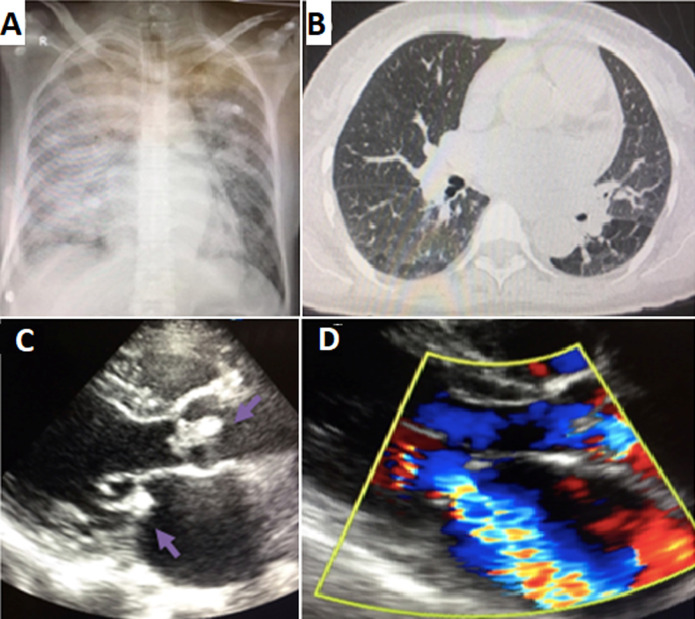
clinical case 2; A) chest X-ray showed a bilateral diffuse alveolar syndrome and cardiomegaly; B) thoracic CT scan a cardiomegaly with the presence of peripheral, central and bilateral ground glass lesion; C) transthoracic echocardiogram: parasternal long axe view showing a vegetation in the aortic and mitral valve (arrows); D) Doppler signal showing mitral regurgitation
